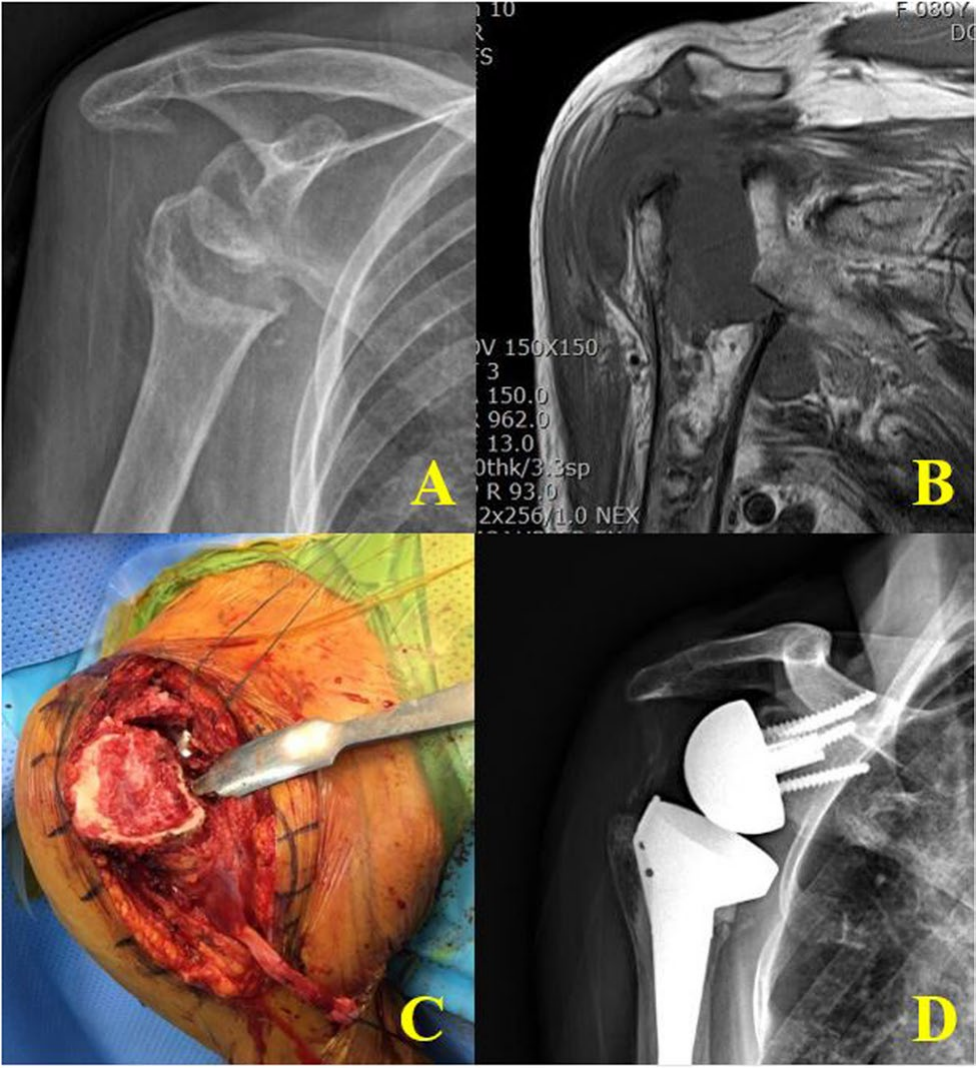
Representative findings in the late stage of a three-stage classification system for RPDA: A Anteroposterior radiograph of the right shoulder showing humeral head collapse, flattening, and osteolytic changes with severe osteopenia. B T1-weighted coronal MRI demonstrating an irreparable rotator cuff tear and significant joint effusion around the humeral head, with relative preservation of the glenoid. C Intraoperative image revealing massive bone loss with destruction of the humeral head extending to the humeral neck. D Postoperative radiograph showing reverse total shoulder arthroplasty
